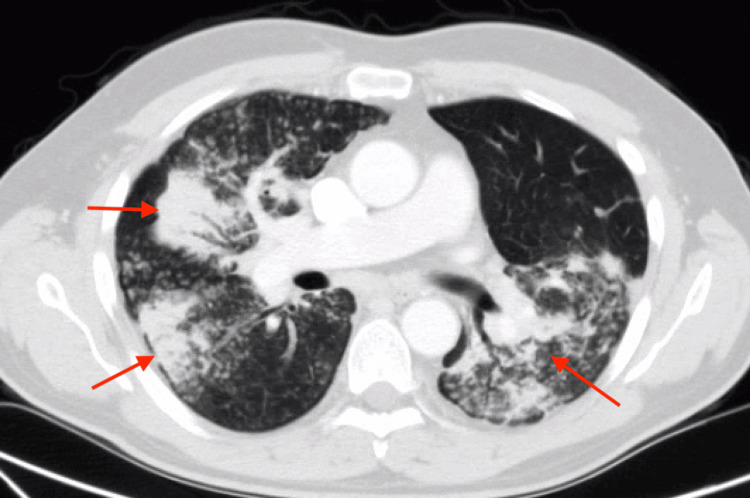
Transverse CT chest view at admission
